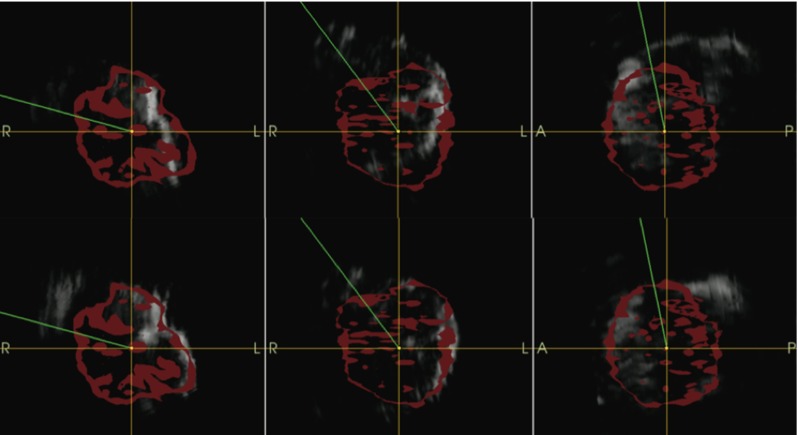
Manual shift correction to determine the position deviation between computed tomography (CT) and ultrasound (US) volumes. Reconstructed US volumes (*gray*) were moved manually to the corresponding surface model segmented from CT (*red*). The optimal alignment was then determined in 2D axial, coronal, and sagittal (ACS) planes. *Top row* ACS planes before manual correction. *Bottom row* ACS planes after manual correction
